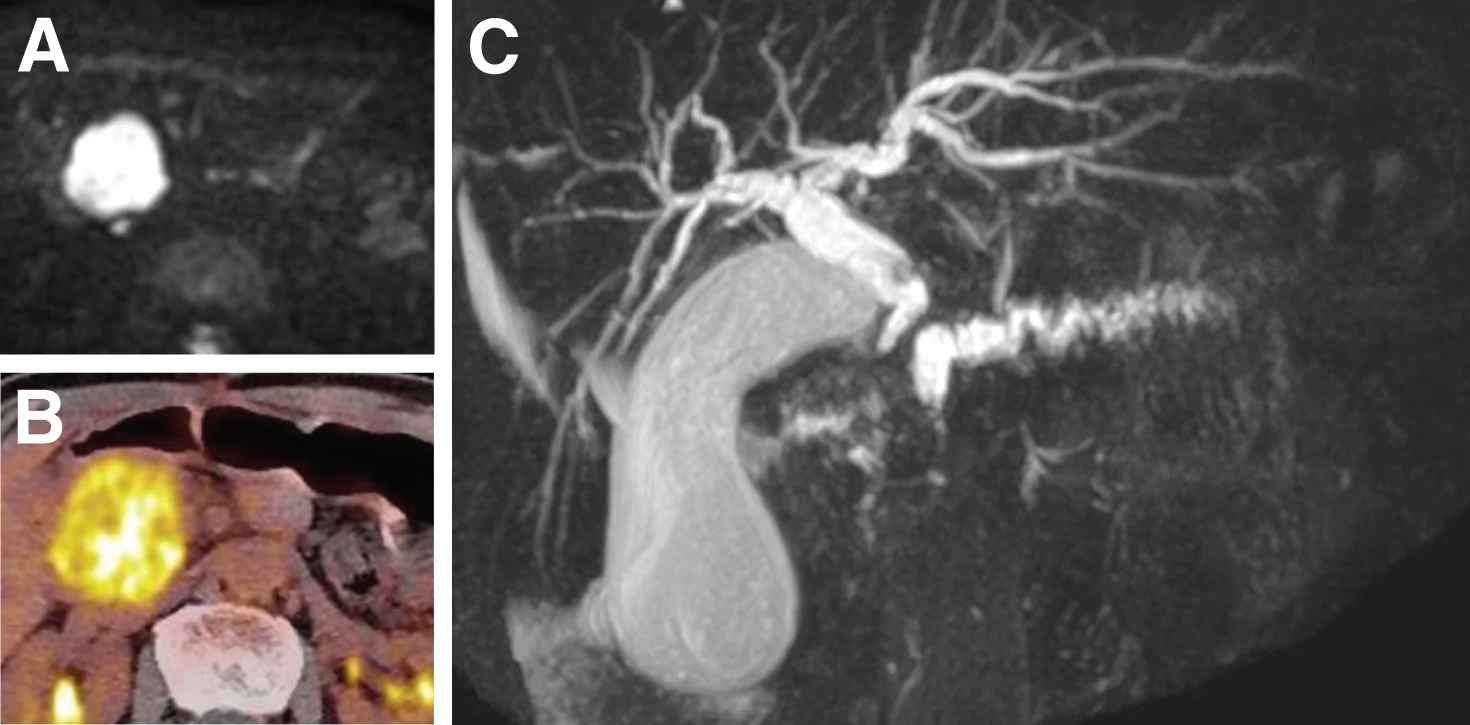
Multimodal imaging findings. (A) Diffusion-weighted MRI showing high signal intensity in the pancreatic head mass. (B) FDG-PET after systemic chemotherapy revealing abnormal uptake in the pancreatic head lesion (SUVmax 6.58). (C) MRCP shows a dilated main pancreatic duct with an abrupt interruption. FDG, ^18^F-fluorodeoxyglucose; SUVmax, maximum standardized uptake value; MRCP, magnetic resonance cholangiopancreatography
